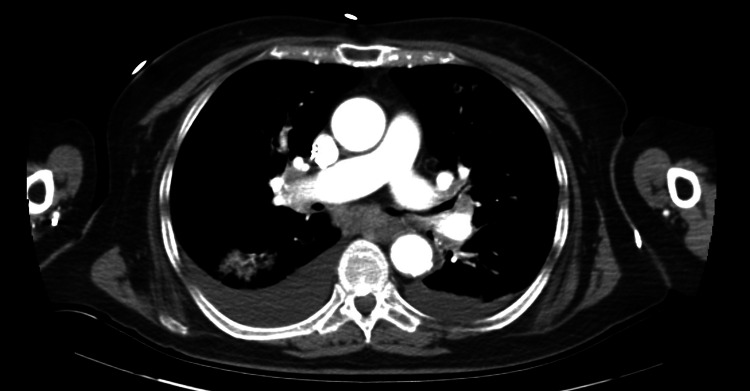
CT of the chest, abdomen, and pelvis Computed tomography (CT) of the chest, abdomen, and pelvis revealed small to moderate right and small left pleural effusions, pulmonary edema, multifocal pneumonia, and atherosclerosis.
